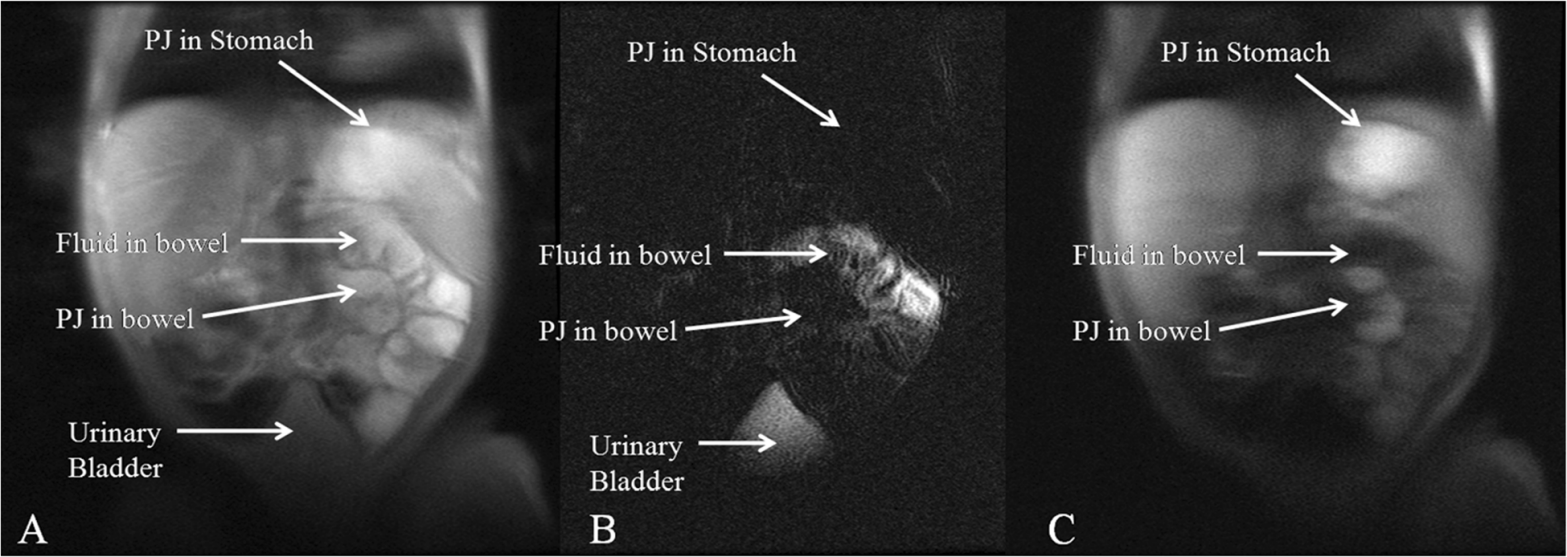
Coronal IR-SSFSE of neonatal GI tract. A 2 week old 3 kg male infant underwent MRI following PJ administration. A. Conventional SSFSE (TR 2500 ms) imaging. It is not possible to differentiate between PJ in the stomach and proximal small bowel from fluid already in the small bowel on conventional T2w imaging. B. T_2_w hydrographic SSFSE image (TR of 4000 ms) demonstrating location of pre-existing bowel fluid. The short T_2_ PJ in the stomach and proximal bowel is low signal. C. IR-SSFSE PDw (TI = 1000 ms) that nulls the signal from pre-existing bowel fluid, and demonstrates PJ as high signal in stomach and proximal bowel.
